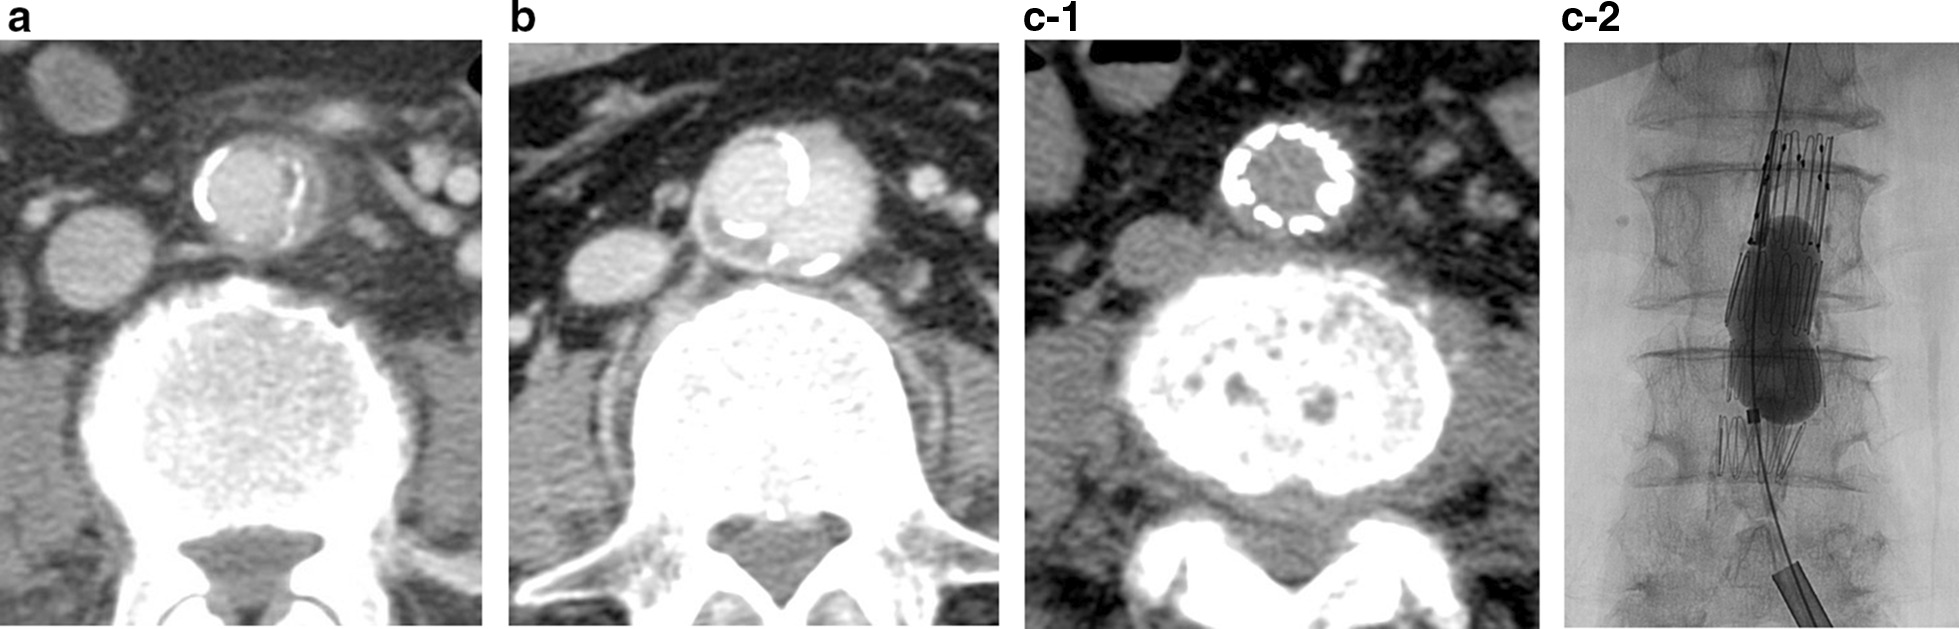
Images of the aortic aneurysm. a Initial computed tomography (CT) image of the abdomen/pelvis, demonstrating a 20-mm abdominal aortic aneurysm, with evidence of inflammatory changes, 20 days after prostate biopsy. b Repeat CT image revealing that the abdominal aortic aneurysm diameter has increased to 30 mm, 64 days after the prostate biopsy. c CT and angiography showing the successful exclusion of the aortic rupture by endovascular stent grafting
